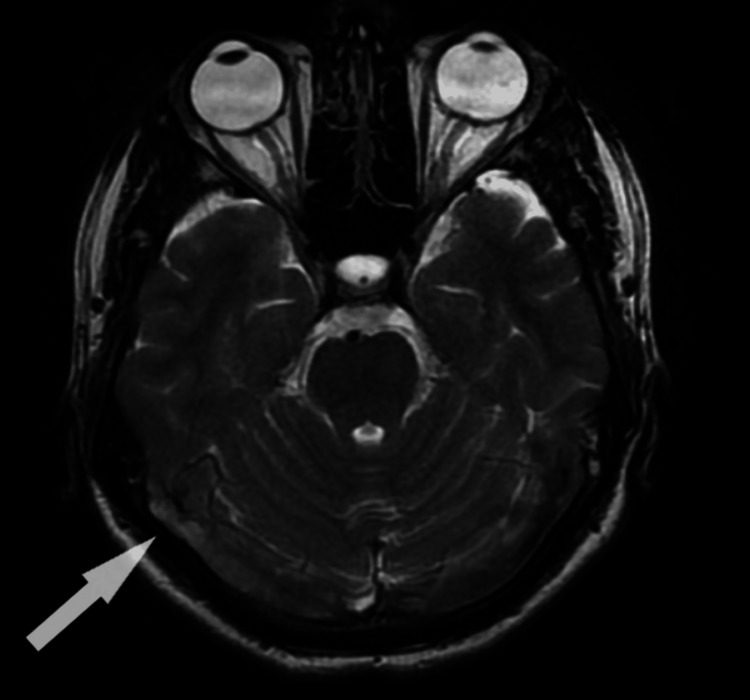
MRI Brain showing hypointense lesion in T2 weighted image (arrow)
